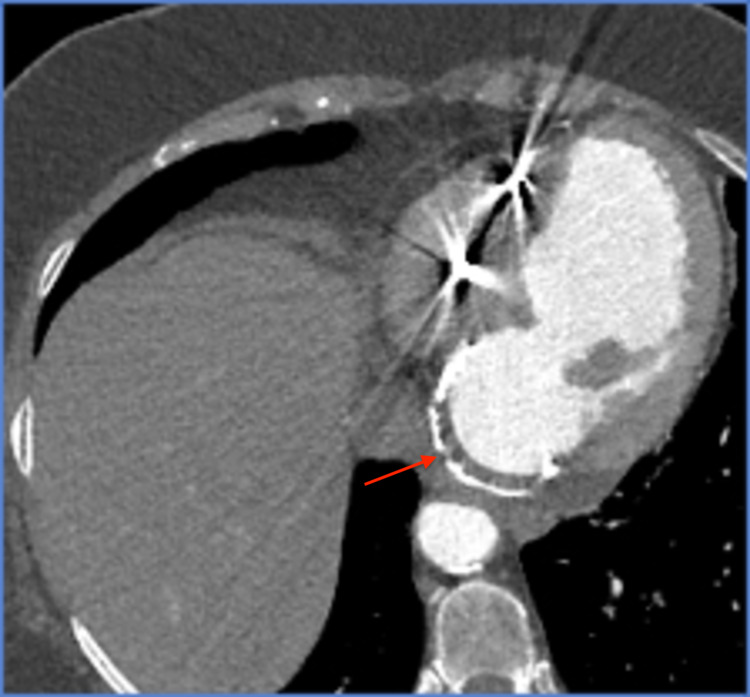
Cardiac computed tomography with angiography showing a large 46 x 45 x 47 mm infero-basal aneurysm with a chronic thrombus and calcified wall.
